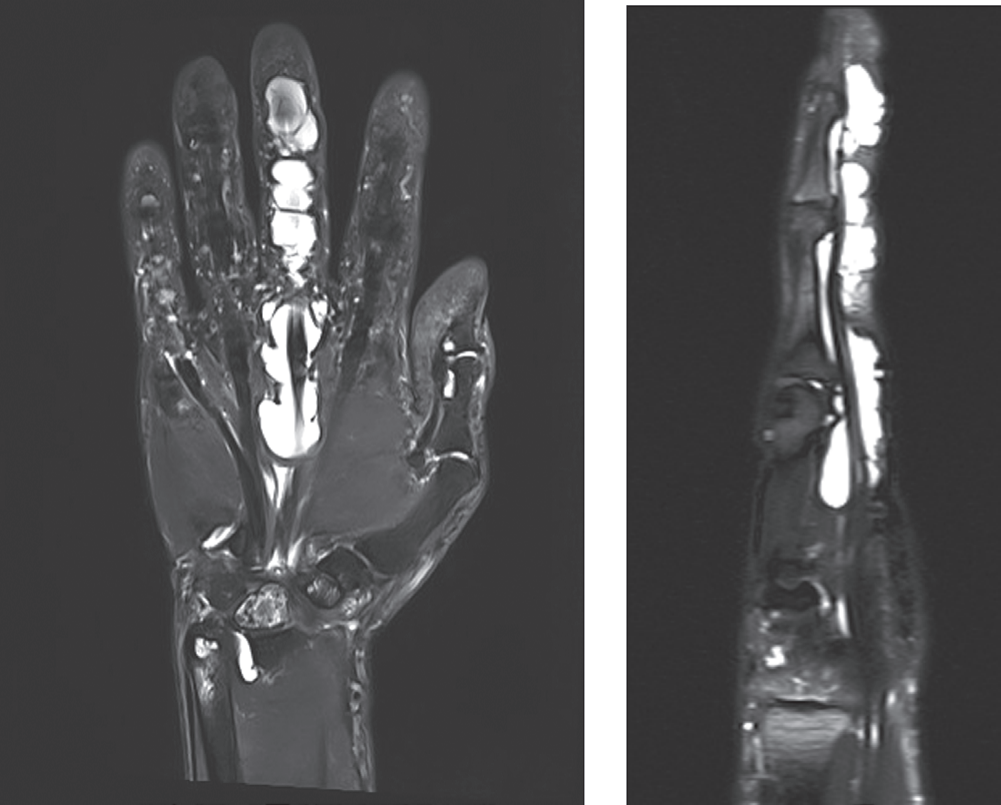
Coronal (A) and sagittal (B) T2-weighted MRI of the left hand revealing severe fluid collection in the flexor sheath, from the metacarpal body to the DIP joint.
